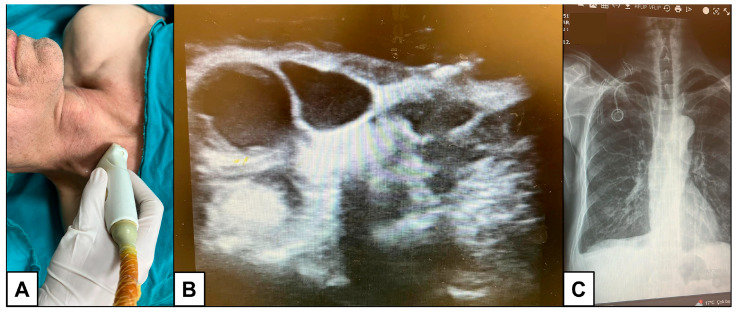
Ultrasound-guided right internal jugular vein access and post-procedural catheter confirmation after TIVAP implantation. (A) Clinical view showing patient positioning and ultrasound probe placement over the right cervical region to identify the internal jugular vein before venous puncture. (B) Transverse ultrasonographic image of the neck demonstrating the target internal jugular vein and the adjacent carotid artery during real-time vascular assessment prior to cannulation. (C) Post-procedural chest radiograph showing the implanted totally implantable venous access port (TIVAP) and catheter, with the tip located in the central venous system at the superior vena cava/cavoatrial junction.
